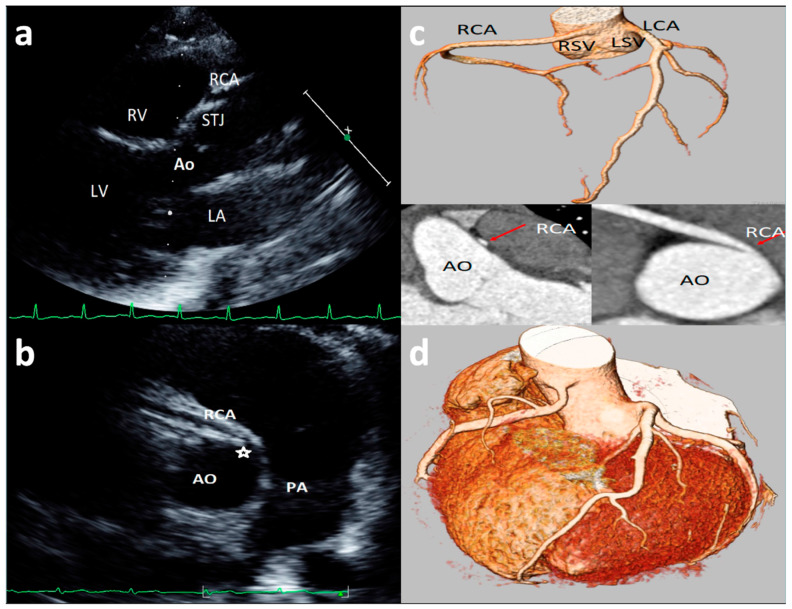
Asymptomatic 17-year-old boy evaluated for the presence of two premature ventricular beats at peak of stress test in routine preparticipation evaluation for sports competition. Parasternal long-axis view showing right coronary artery (RCA) high take-off coronary artery above the sinus of Valsalva (a). The acute angulation origin is clearly visualized in short-axis view (b) and was confirmed at CT (c). After CT confirmation of the defect, a myocardial scintigraphy was performed revealing a small ischemia (8%) in the RCA territory at peak. We advised sport restriction. Ao = aorta, LCA = left common coronary artery, RCA = right coronary artery, LSV = left sinus of Valsalva, RSV = right sinus of Valsalva. (d) is an example of 73-year-old man showing symptoms of dyspnea. 3D-Volume Rendering CCTA showing the right dominant coronary artery (RCA) high take-off two centimeters distal to the sinutubular junction at the level of the anterior ascending aortic wall.
